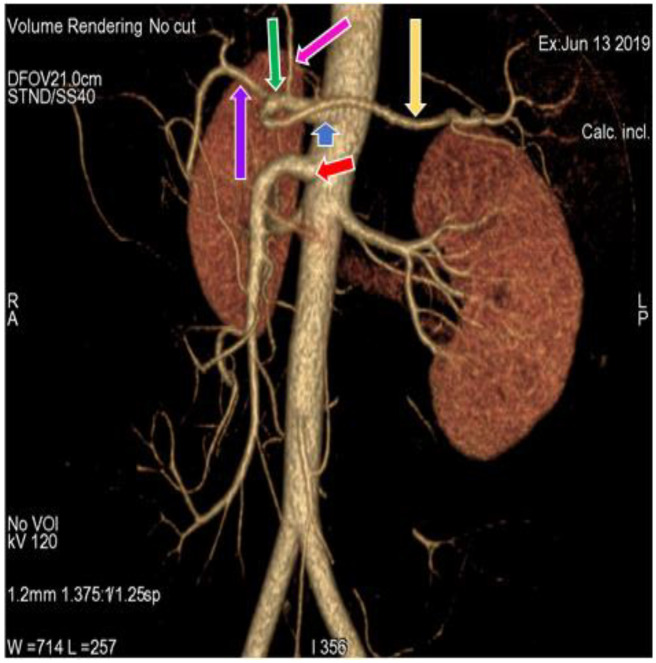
3D-VR image of (64 row scanner CT angiography) abdominal region showing superior mesenteric artery (red arrow) and division of Celiac trunk (blue arrow) into left gastric artery (pink arrow) and hepatosplenic trunk (green arrow). Hepatosplenic trunk further divides into common hepatic artery (purple arrow) and splenic artery (yellow arrow).
